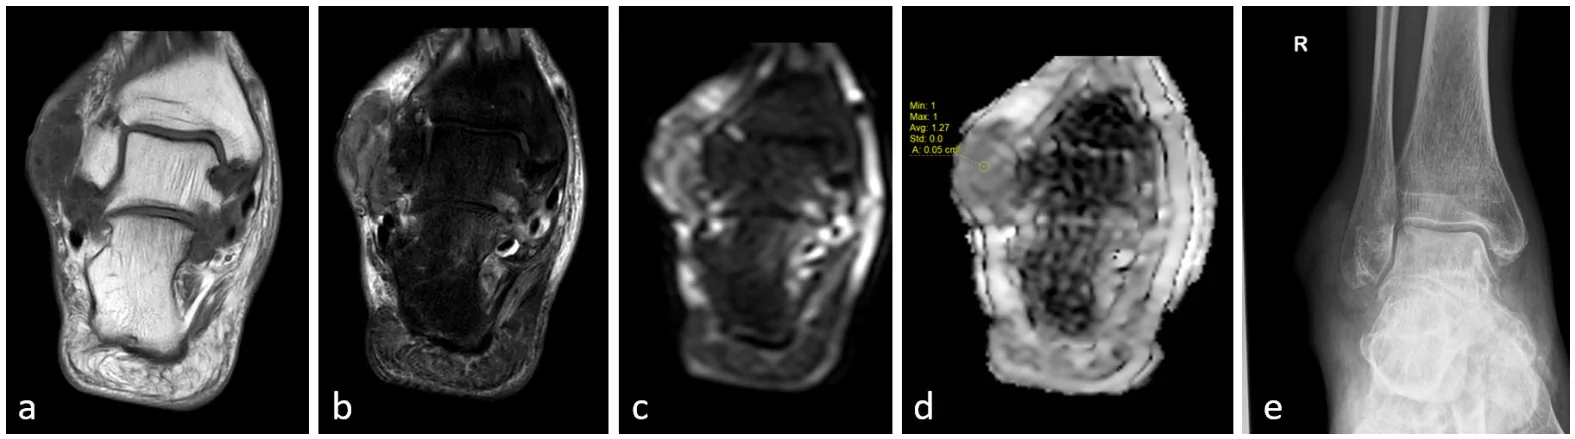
Coronal MRI and radiograph of the ankle demonstrating a gouty tophus. The lesion presents as a lobulated mass on the lateral aspect of the ankle, appearing as predominantly T1-weighted hypointense (a) and fat-suppressed T2-weighted hypointense (b). Importantly, whilst the ADC map (d) demonstrates apparently low signal, suggesting restricted diffusion, DWI (c) shows no corresponding hyperintensity—a recognised pitfall in which spuriously low ADC signal in the absence of DWI hyperintensity may be erroneously interpreted as true restricted diffusion. The frontal radiograph (e) demonstrates a lobulated soft tissue swelling with internal foci of calcification, characteristic of gouty tophi.
